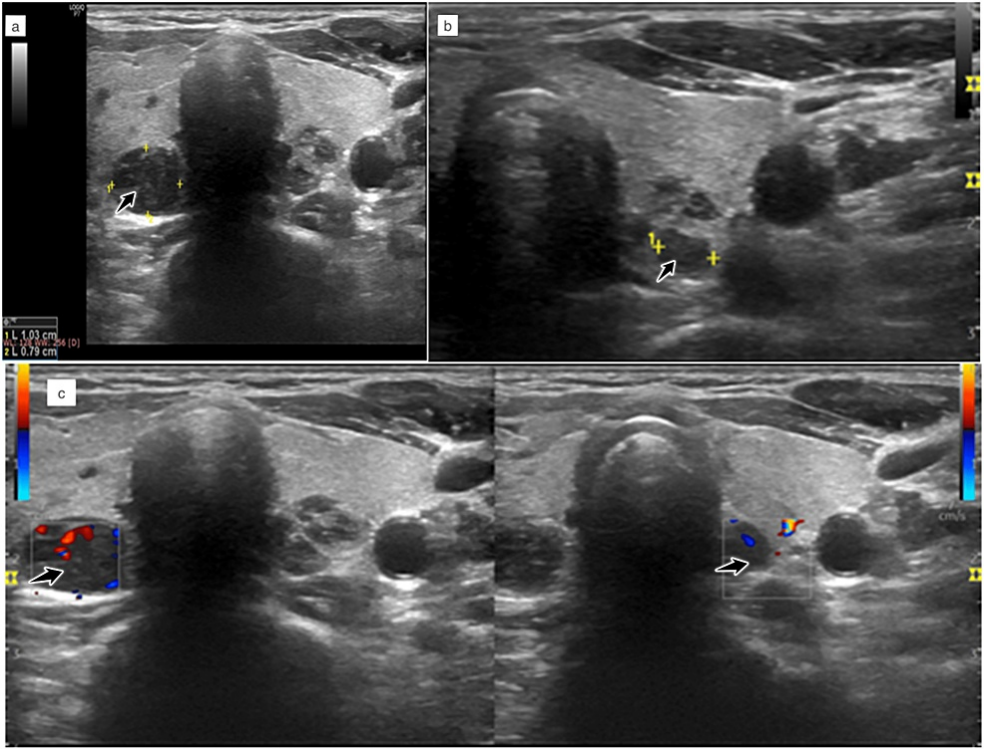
(a-c) Greyscale and color Doppler neck ultrasound (transverse views). Hypoechoic lesions on the posterior aspect of both lobes of thyroid with vascularity (marked with arrow).
